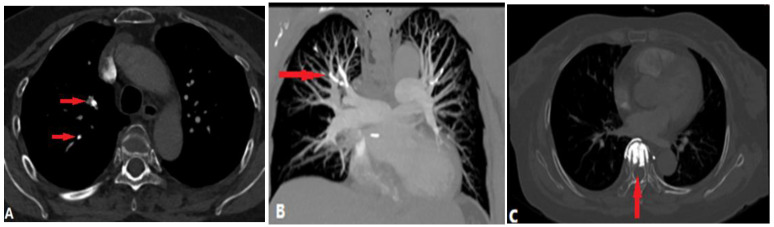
Pulmonary cement embolism. (A,B): Axial CTPA and coronal MIP show the cement embolus (high density-arrows) in the pulmonary arteries of the upper-right and -left lobes. (C): Cement (arrow) in the vertebral body and the external vertebral venous plexuses. CTPA—computed tomography pulmonary angiography; MIP—maximum-intensity projection.
